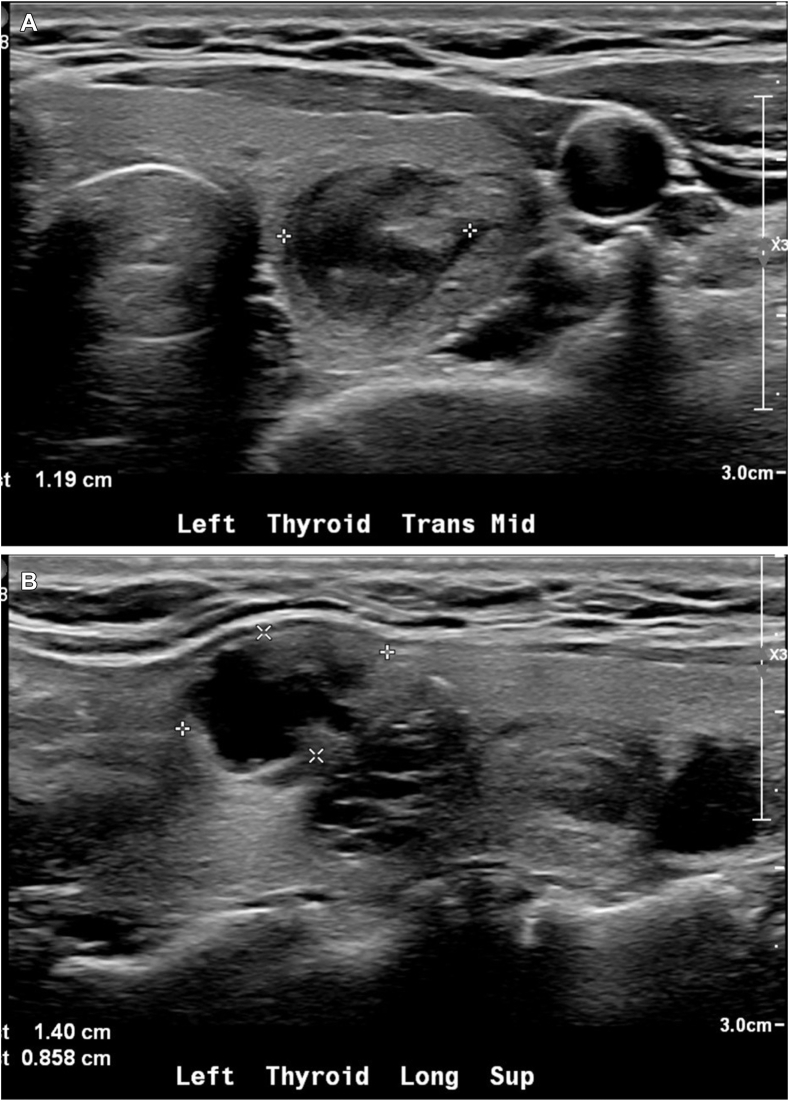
Thyroid ultrasound images of *left* mid thyroid solid and hypoechoic nodule. The nodule measured 1.3 × 1.2 × 1.2 cm and was TI-RADS 4. It did have internal vascular flow. *A*, Antero-posterior view; *B*, Longitudinal view.
